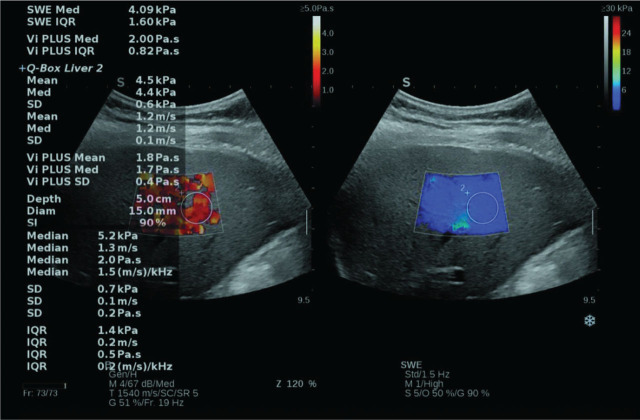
The image of a Vi.PLUS measurement, which was performed in a NAFLD patient. The viscosity is displayed in the left part of the image. Colors close to yellow white are indicative of high viscosity, while red means low viscosity. Numeric Vi.PLUS results (expressed in Pa.S) are shown on the left side of the image. NAFLD, non-alcoholic fatty liver disease; SD, standard deviation; SI, stability index; Vi.PLUS, Viscosity plane-wave ultrasound.
